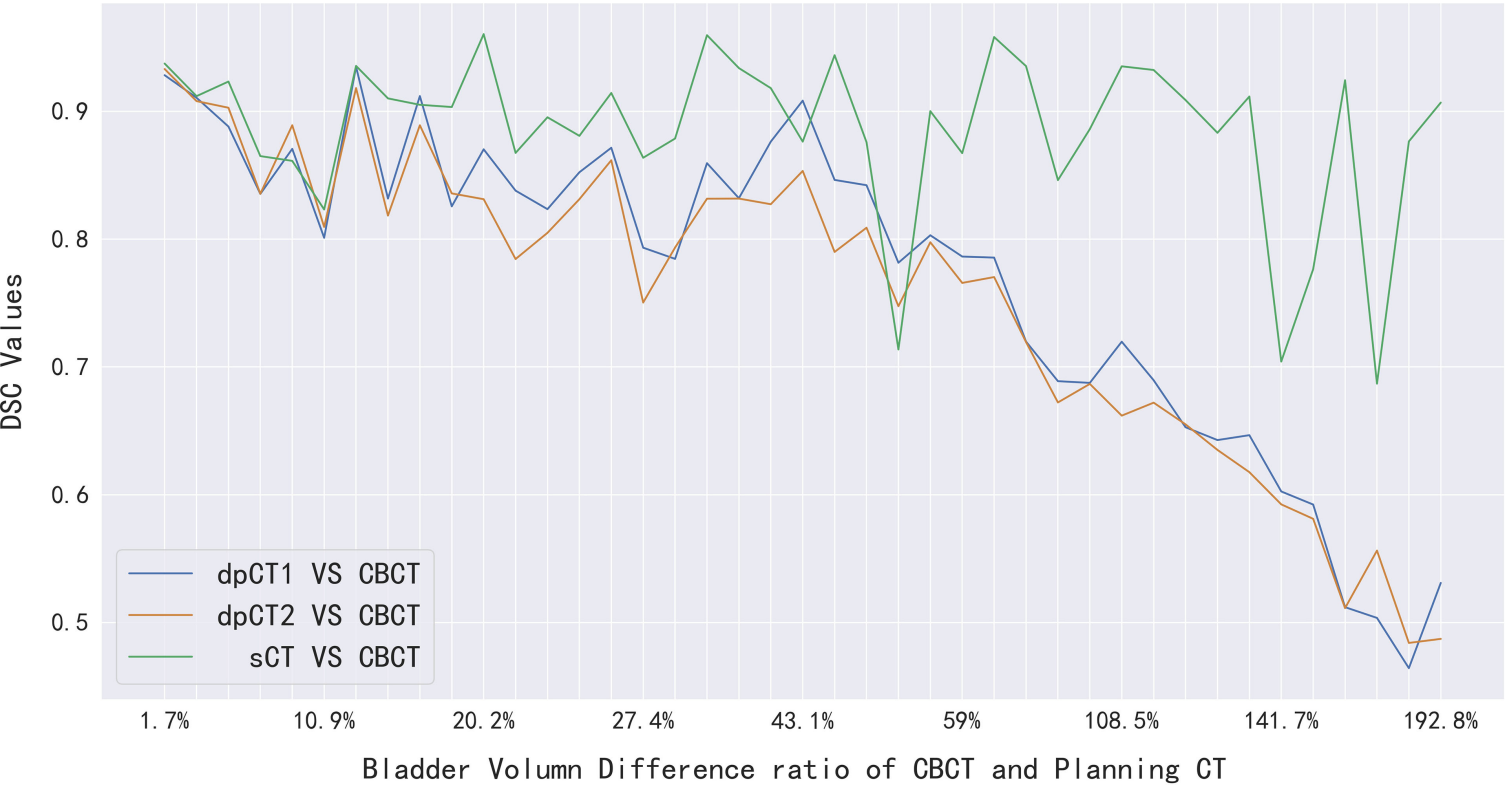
The effect of bladder volume difference on registration and sCT accuracy. dpCT1, deformed planning CT with Elastix; dpCT2, deformed planning CT with MSnet.
